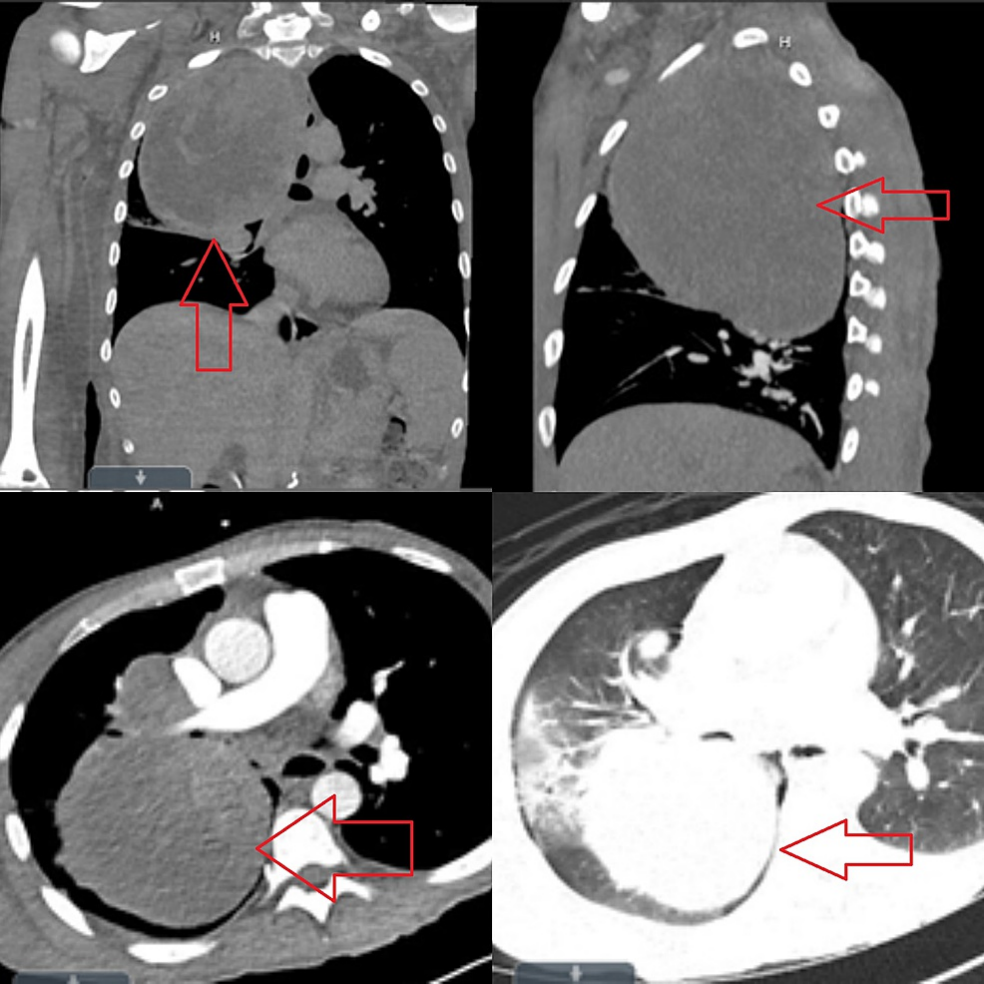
CT scan of the thorax with contrast. An irregular, thick-walled, 14-cm lung mass occupying the middle and upper hemithorax and compressing the right mainstem bronchus. The image also shows a filling defect in the left atrium, right atrial appendage, and right pulmonary vein. CT, computed tomography
